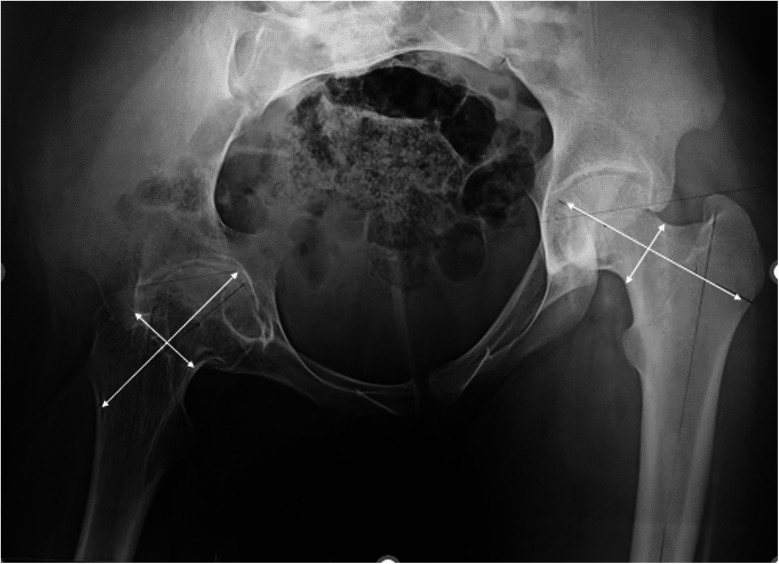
Pelvic radiograph of a 17-year-old girl with enthesitis-related arthritis JIA showing hip destruction with growth abnormalities assessed by asymmetric length and width femoral measurements.
